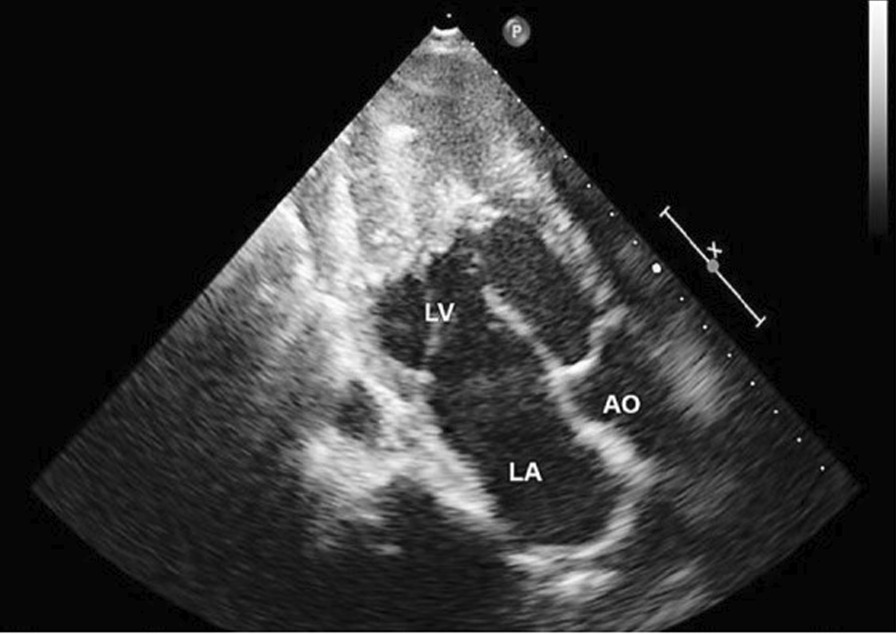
Echocardiogram revealing increased thickness in the septum (17 mm) and free left ventricular wall (15 mm), obliteration of the left ventricular apex and inflow tract, and mitral valve regurgitation. *LV* left ventricle, *LA* left atrium, *AO* aorta
